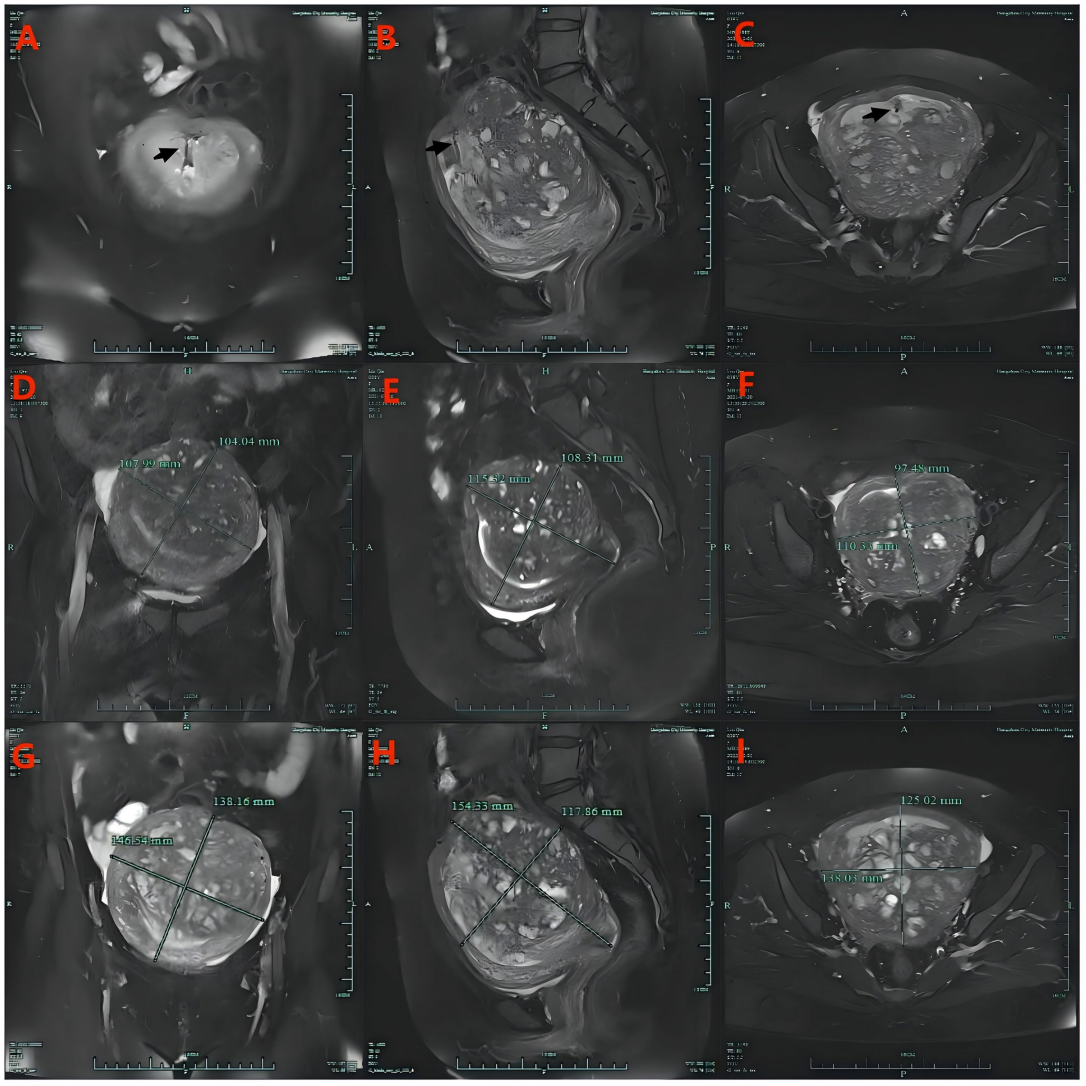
The Mirena is visible in A–C (black arrow). (D–F) Are magnetic resonance images of the uterus in coronal, sagittal, and transverse planes, respectively, before Mirena fixation. (G–I) Are the magnetic resonance images of the uterus in coronal, sagittal, and transverse planes, respectively, 1 year after Mirena fixation.
